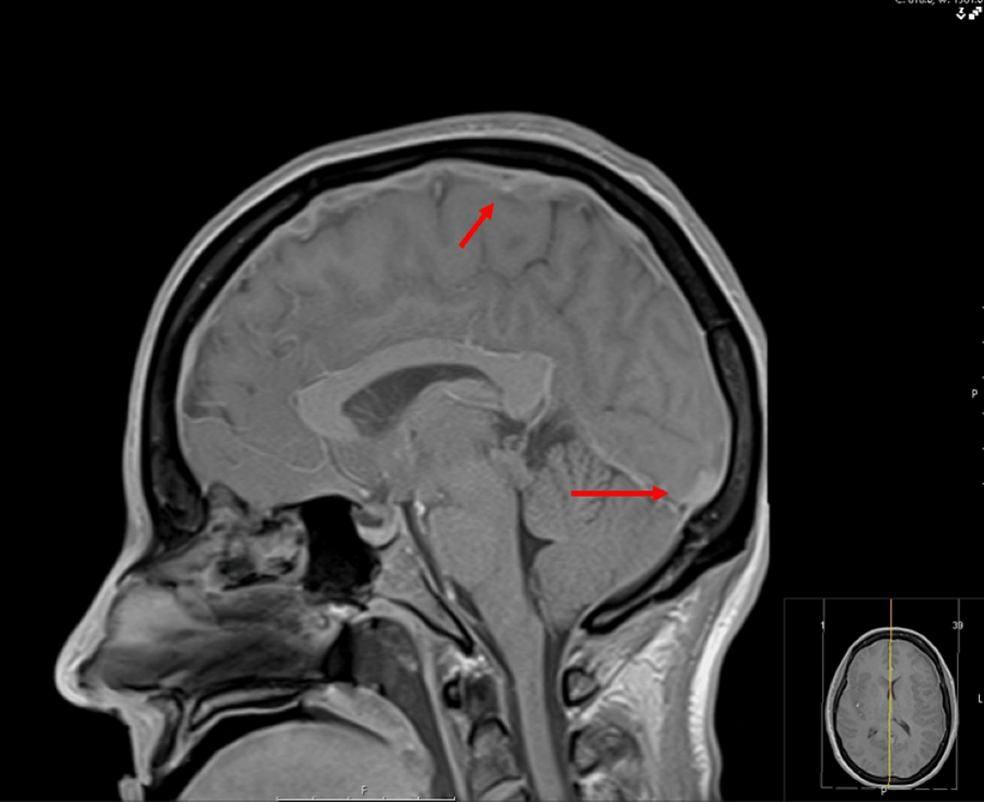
T1-weighted MRI shows sagittal vein thrombosis (sagittal plane) MRI: magnetic resonance imaging.
